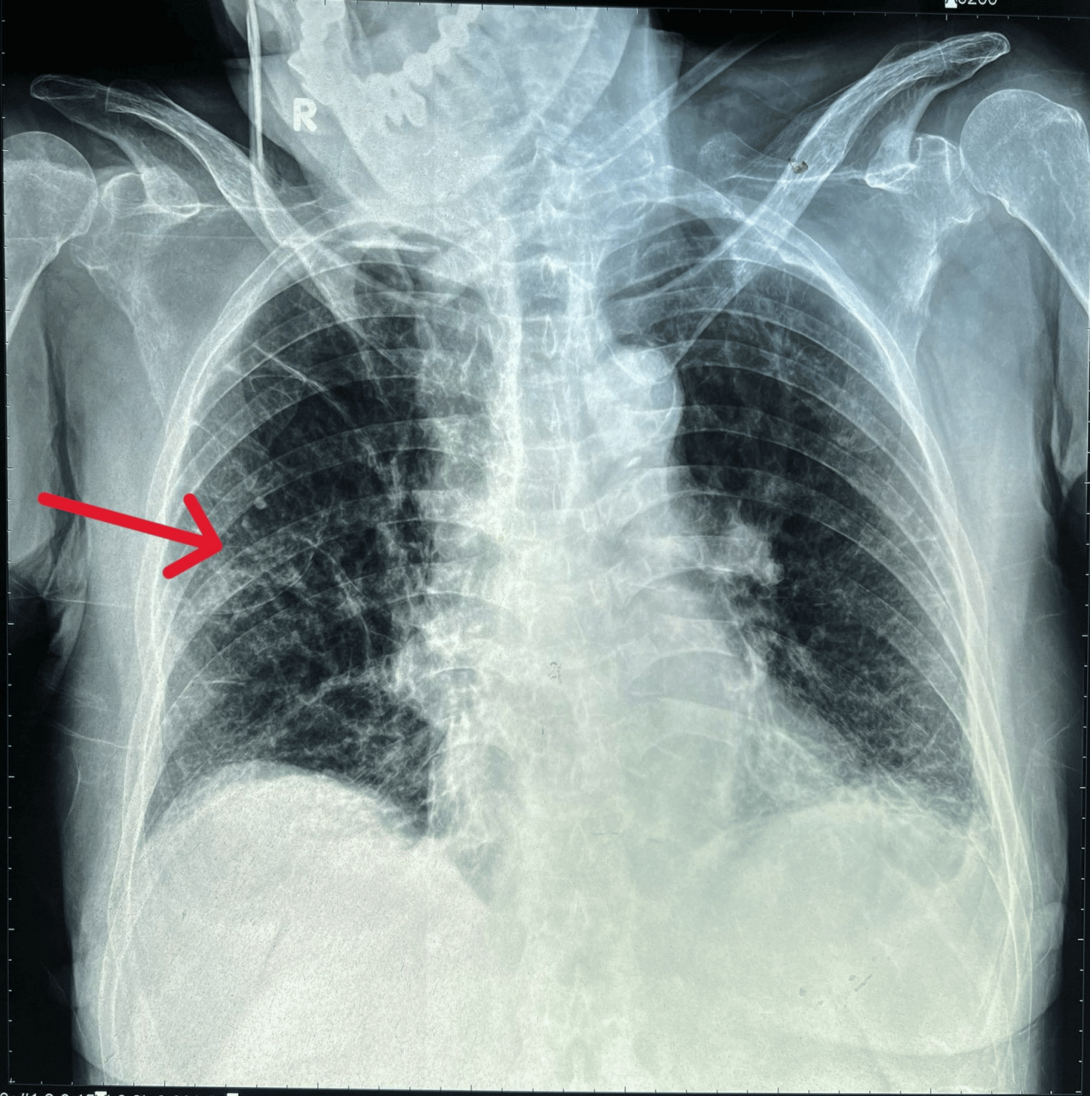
Chest X-ray revealed infiltrates in the middle third of the right lung (red arrow) and diffuse fibrosis throughout both lungs
